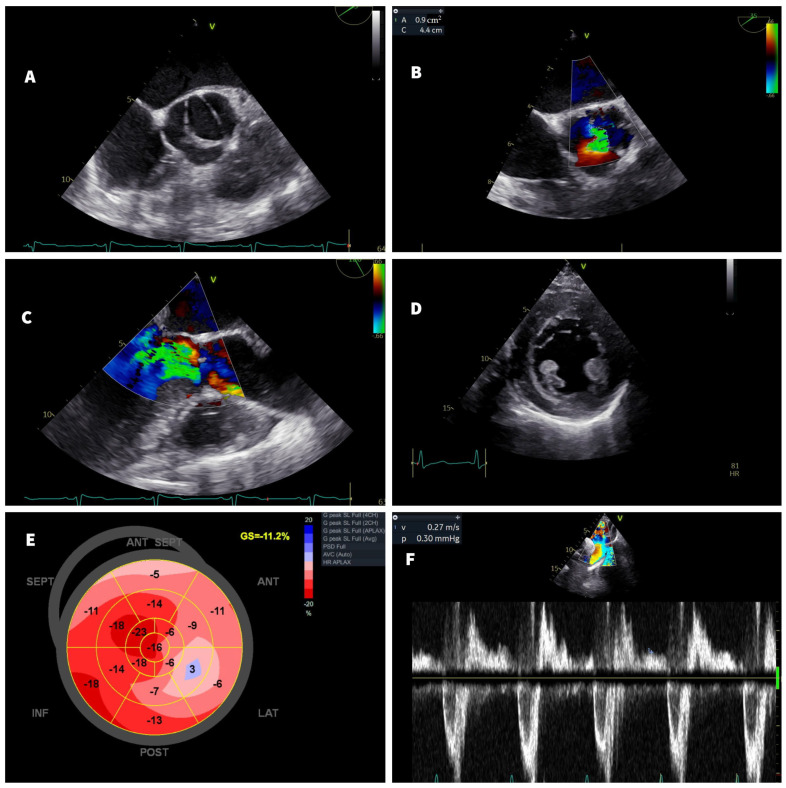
Transthoracic and transesophageal echocardiography. (A,B) Mid-esophageal short-axis view- bicuspid aortic valve with the fusion of the right and noncoronary cusp and color Doppler of the aortic valve with severe regurgitation. (C) Mid-esophageal long-axis view- color Doppler showing severe eccentric aortic regurgitation jet, with the jet width/left ventricle outflow tract (LVOT) diameter >90%. (D) Parasternal short-axis view- severe concentric left ventricular hypertrophy. (E) Bull’s-eye plot of longitudinal strain showing the reduced global longitudinal strain. (F) Pulsed Doppler recording within the descending aorta demonstrates flow reversal throughout the diastole, with an end diastolic flow velocity of 27 cm/s.
